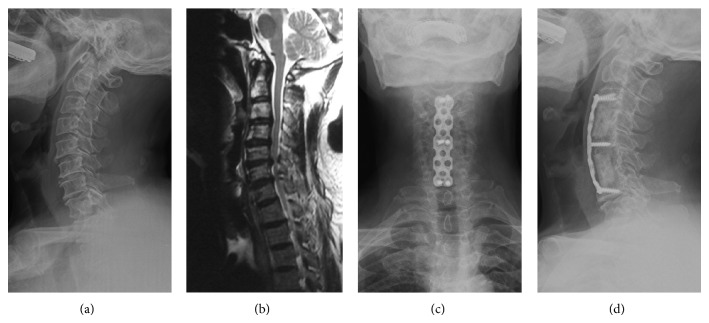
A 65-year-old male patient presented with a 2-year history of pain in the neck and shoulders accompanied by numbness in both hands. Preoperative X-ray and MRI images show proliferation of osteophytes, protrusions of C3/4 and C6/7, and spinal compression caused by hypertrophy of ligamentum flavum. (c) and (d) show the anteroposterior and lateral images on neutral position of the cervical spine after SCF surgery.
